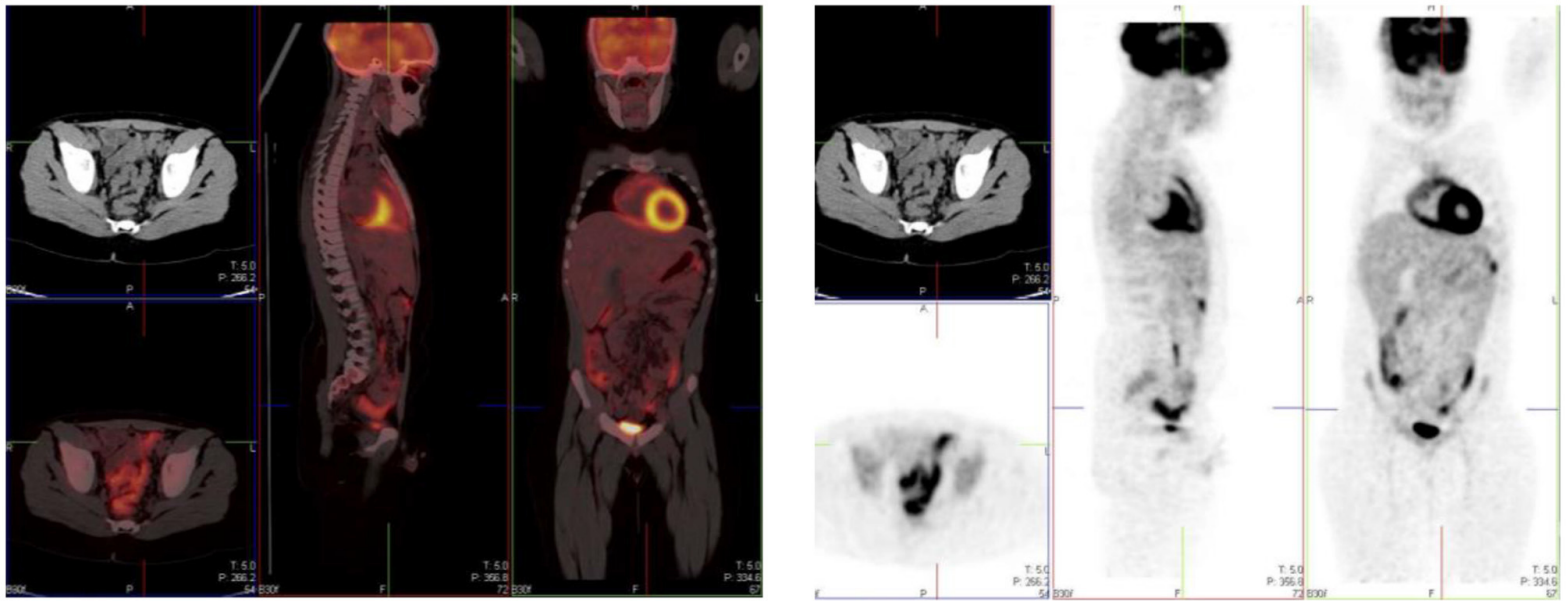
Physiologic uptake of ^18^F-FDG. Axial, sagittal and coronal FDG PET/CT (left) and PET images (right) show physiologic uptake in a 13 year old boy. Normal FDG uptake is seen in the brain, heart, liver, spleen, colon, urinary bladder and the bone marrow. Note the marked intense activity in the brain compared with the activity in the rest of the body.
